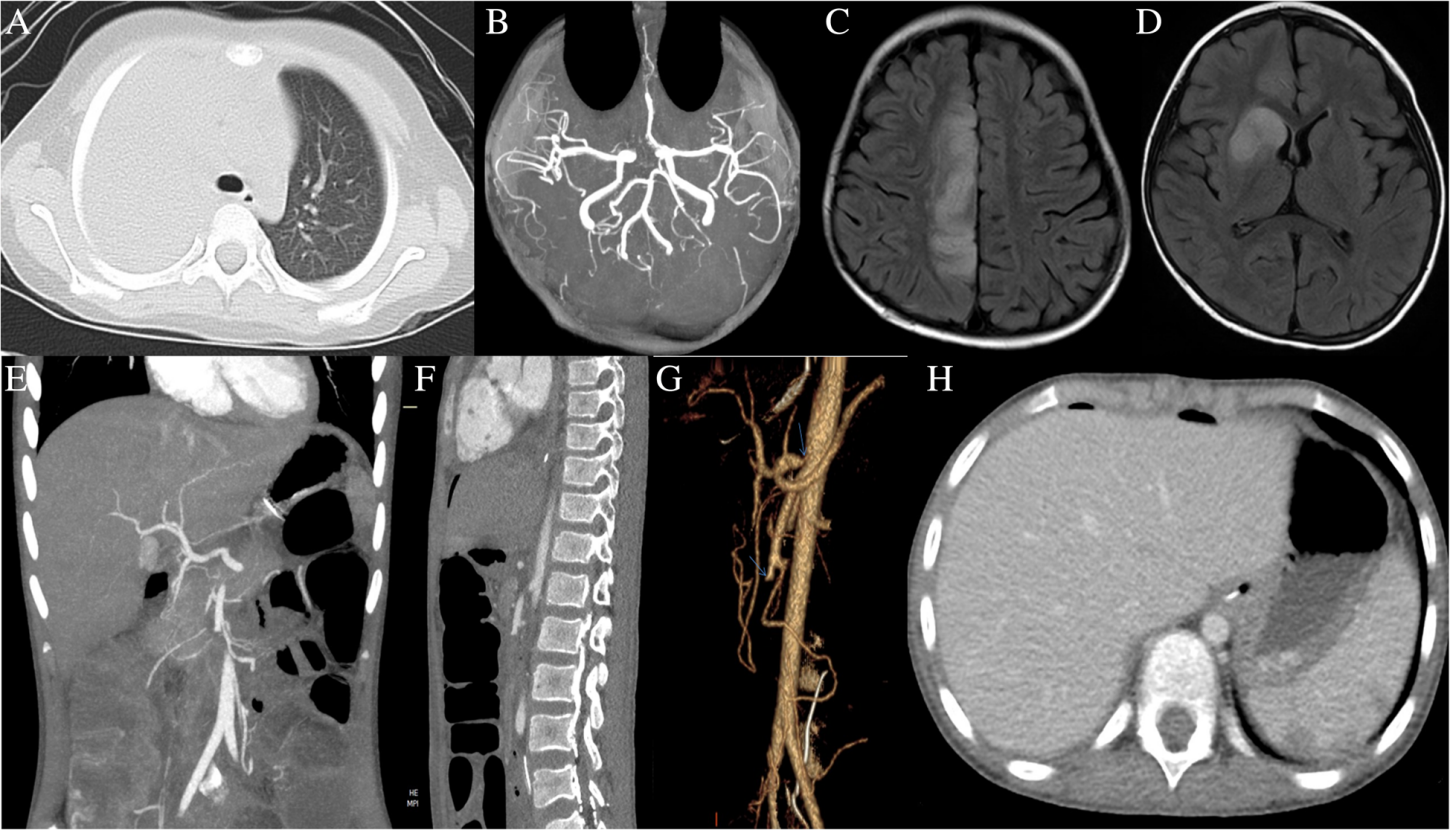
Chest imaging revealed consolidation with high density in the right upper lung (a). 3D-TOF MRA image of the brain didn’t reveal the A1 segment of the right anterior cerebral artery (b), and the T2 FLAIR transverse image showed a patchy high signal in the right parietal cerebral palsy and the right basal ganglia (c, d). Abdominal enhanced CT and 3D vascular reconstruction revealed the superior mesenteric artery thrombosis (e, f, g), and multiple small infarction in the spleen (e, h)
